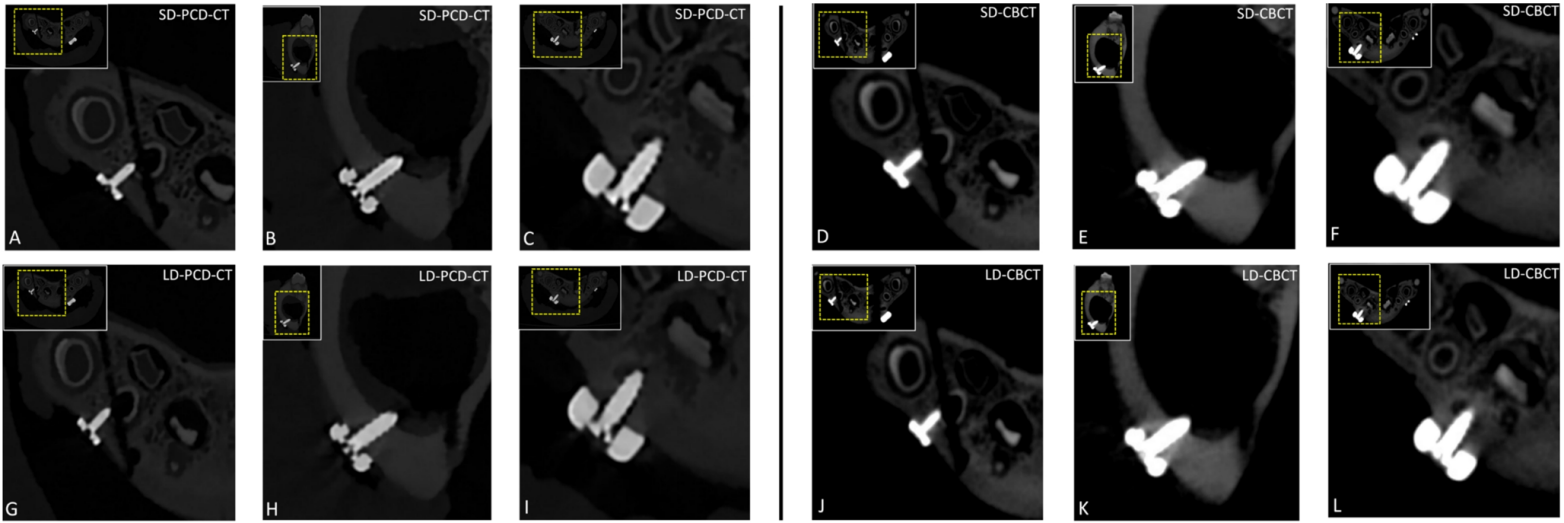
Mandibular fracture fixations using titanium-based microplates and reconstruction plates of varying thicknesses, as well as screws of different lengths and diameters, shown in standard-dose photon-counting computed tomography (PCD-CT) A–C, dose-equivalent cone-beam computed tomography (CBCT) D–F, low-dose PCD-CT G–I, and corresponding dose-matched low-dose CBCT J–L
